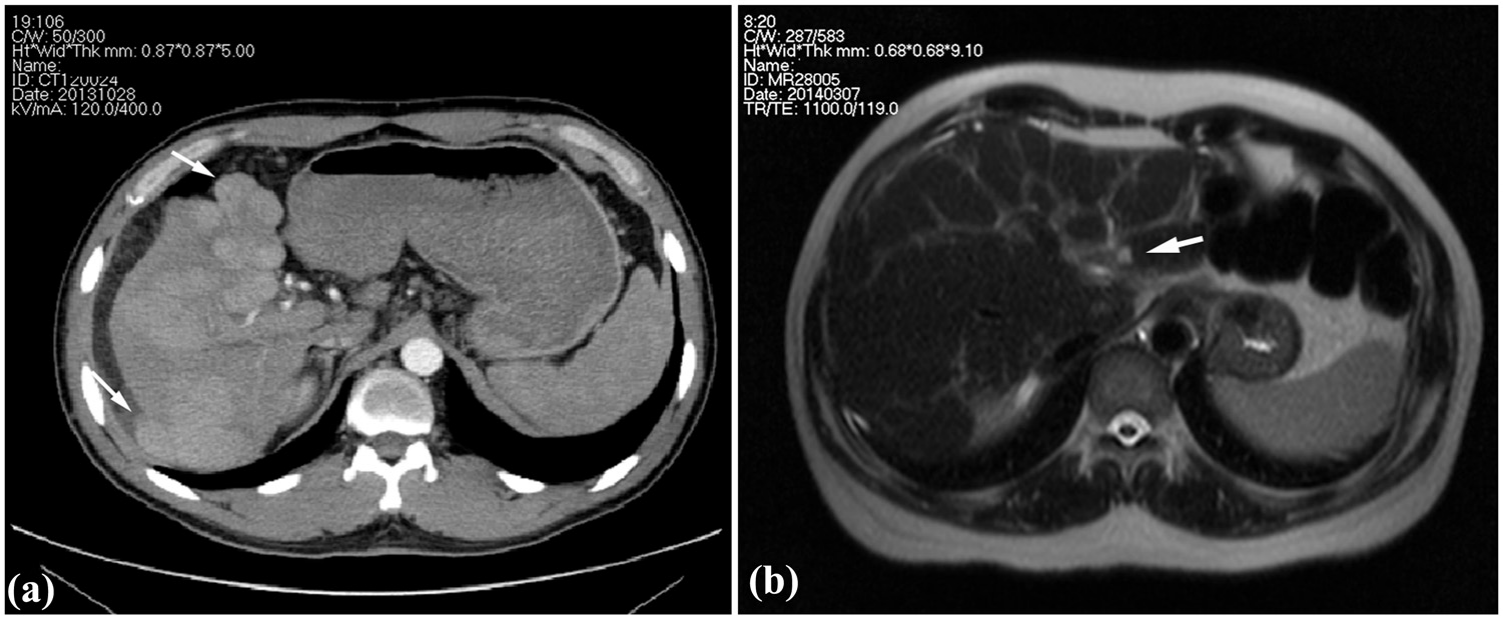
Images of clinical cases. (a) Enhancement CT showing the multiple intrahepatic nodules protruded from the liver surface (arrow) in Case 1 and (b) T2WI of MR showing heterogeneous fibrosis and a nodule in the left inner lobe (arrow) in Case 2.
